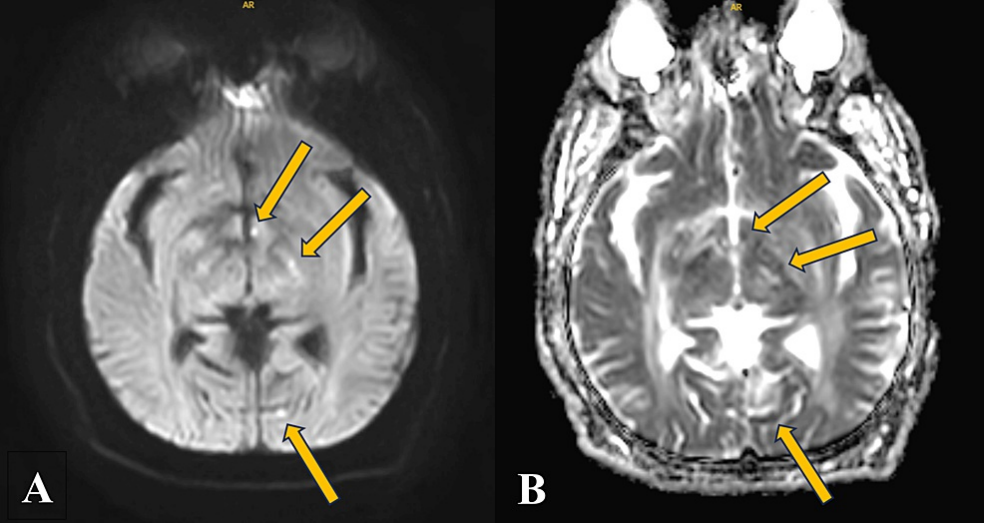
ADC MRI series ADC MRI series revealing multiple punctate hypointensities consistent with ischemic changes. ADC: apparent diffusion coefficient
